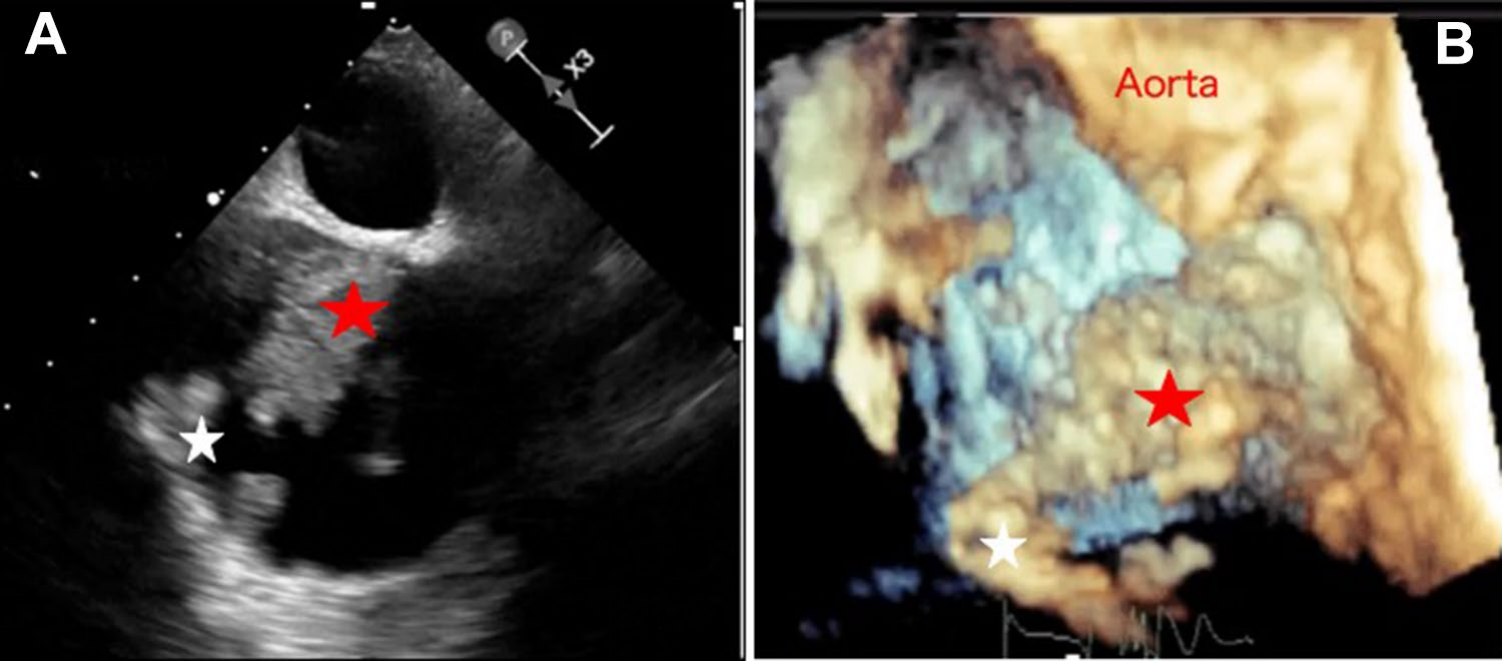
Transesophageal echocardiography (TEE) revealed a tumor adherent to the atrial septum (red star) as well as another 5-mm tumor on the anterior wall of the right atrium (white star) a Image of the right atrial tumors on TEE b Image of the right atrial tumors on three-dimensional TEE
